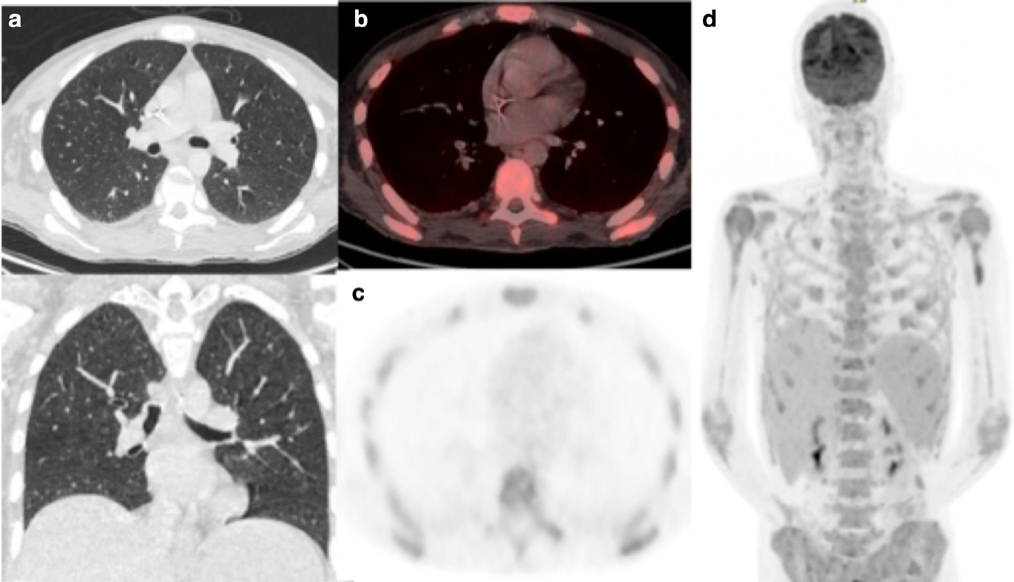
(a) Axial low-dose CT thorax, (b) axial fused thoracic ^18^FDG-PET/CT, (c) ^18^FDG-PET thorax, (d) Maximum-intensity projection. The images demonstrate diffuse marrow uptake. No lung parenchymal change or increased tracer uptake. ^18^FDG-PET, 18-fludeoxyglucose-positron emmision tomography.
